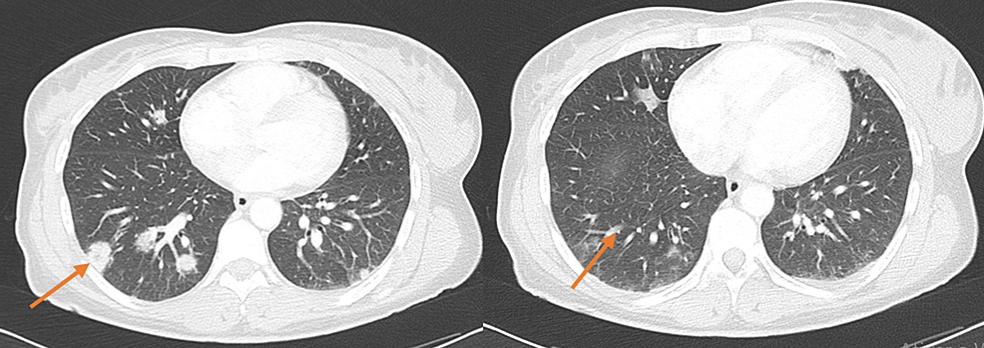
CT on admission showing parenchymal densification (arrows)
